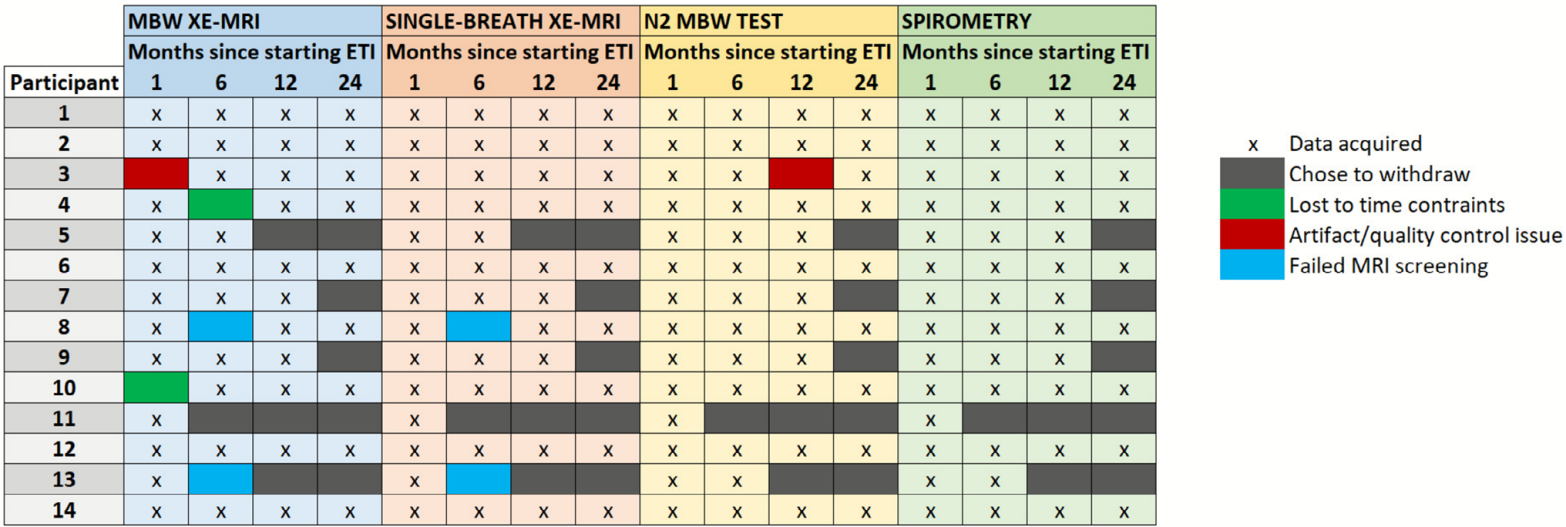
Overview of data availability for multiple‐breath‐washout xenon MRI (MBW Xe‐MRI), single‐breath Xe‐MRI, nitrogen multiple‐breath washout (N_2_ MBW) test, and spirometry across visits at 1, 6, 12, and 24 months following elexacaftor/tezacaftor/ivacaftor (ETI) initiation. Each row represents a participant, with colored cells indicating reasons for missing data (gray, withdrawal; green, lost to time constraints; red, artifact/quality control issue; blue, failed MRI screening) and “*x*” indicating successful data acquisition.
